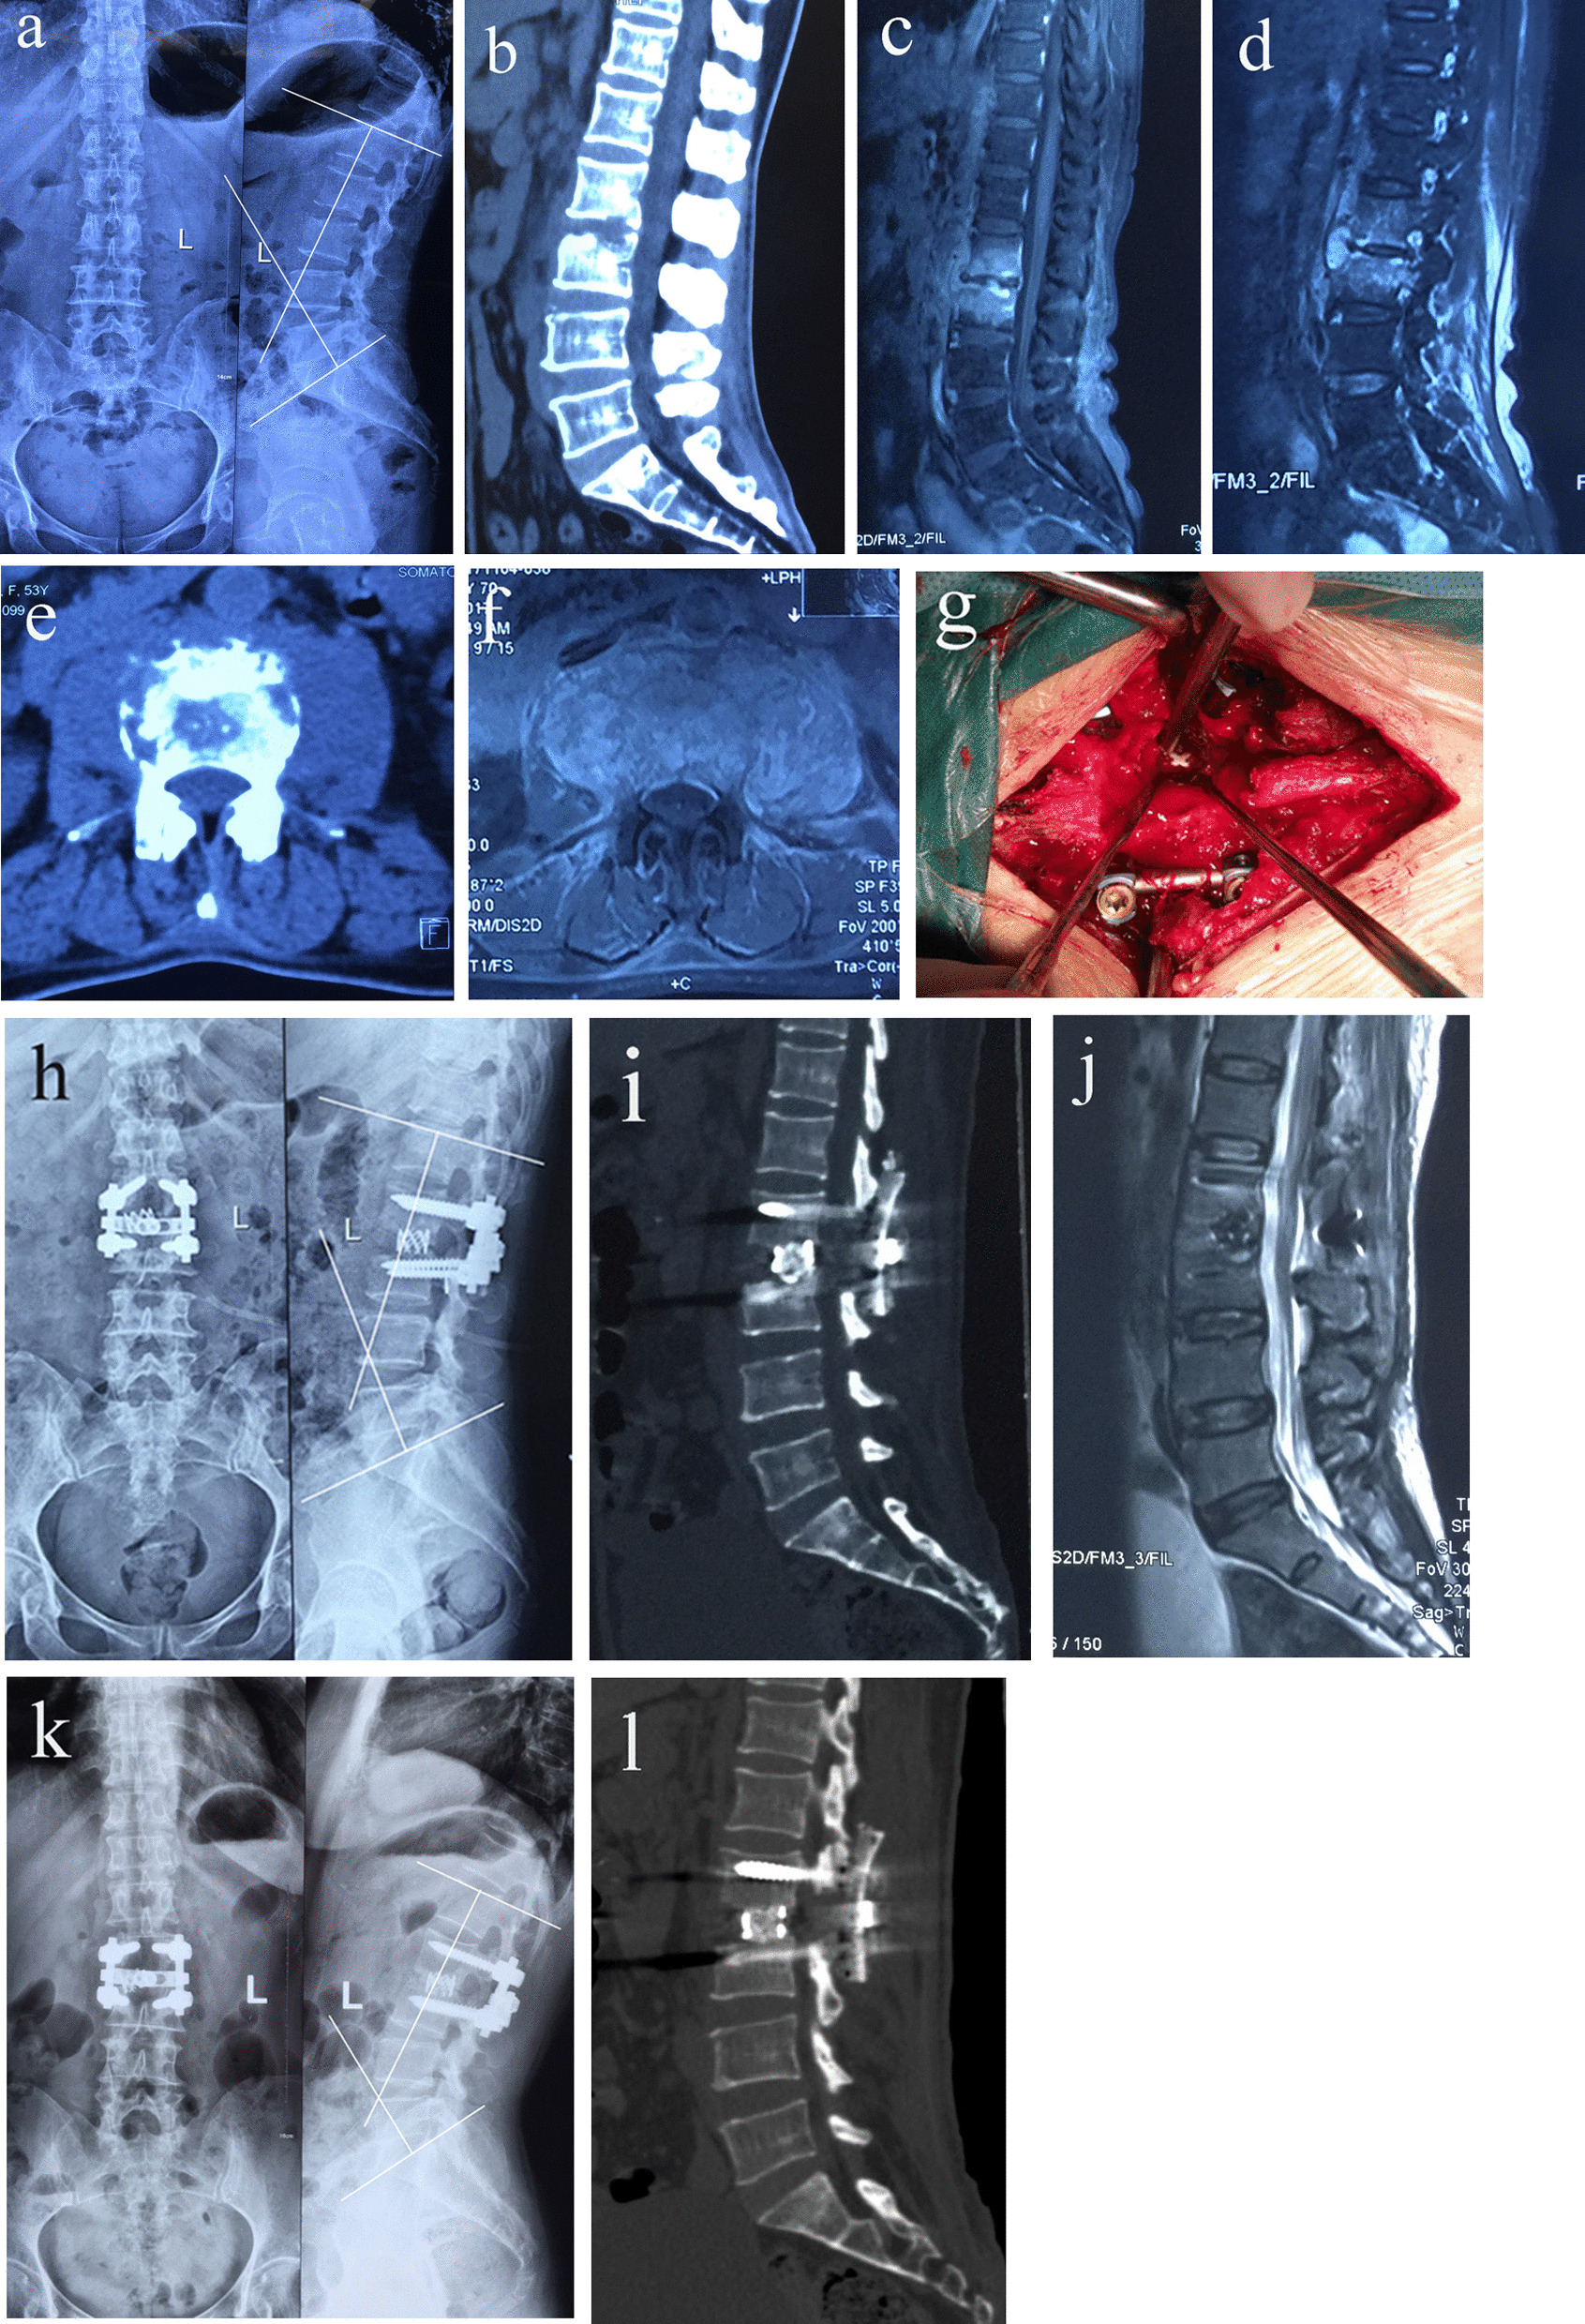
a–f Preoperative radiographs, CT and MRI showed that L2–L3 pyogenic vertebral osteomyelitis and lesion around vertebral body of L2–L3 developed paravertebral abscess with marked bony destruction. g Posterior interbody grafts using titanium mesh cages was applied posterolaterally. h–j Postoperative radiographs showed that posterior interbody graft using titanium mesh cage, posterior single-segmental instrumentation and fusion. Postoperative CT and MRI showed completely resolution of epidural abscess. Interbody graft using titanium mesh cages were placed satisfactorily. k, l Final follow-up radiographs and CT showed good bone fusion
